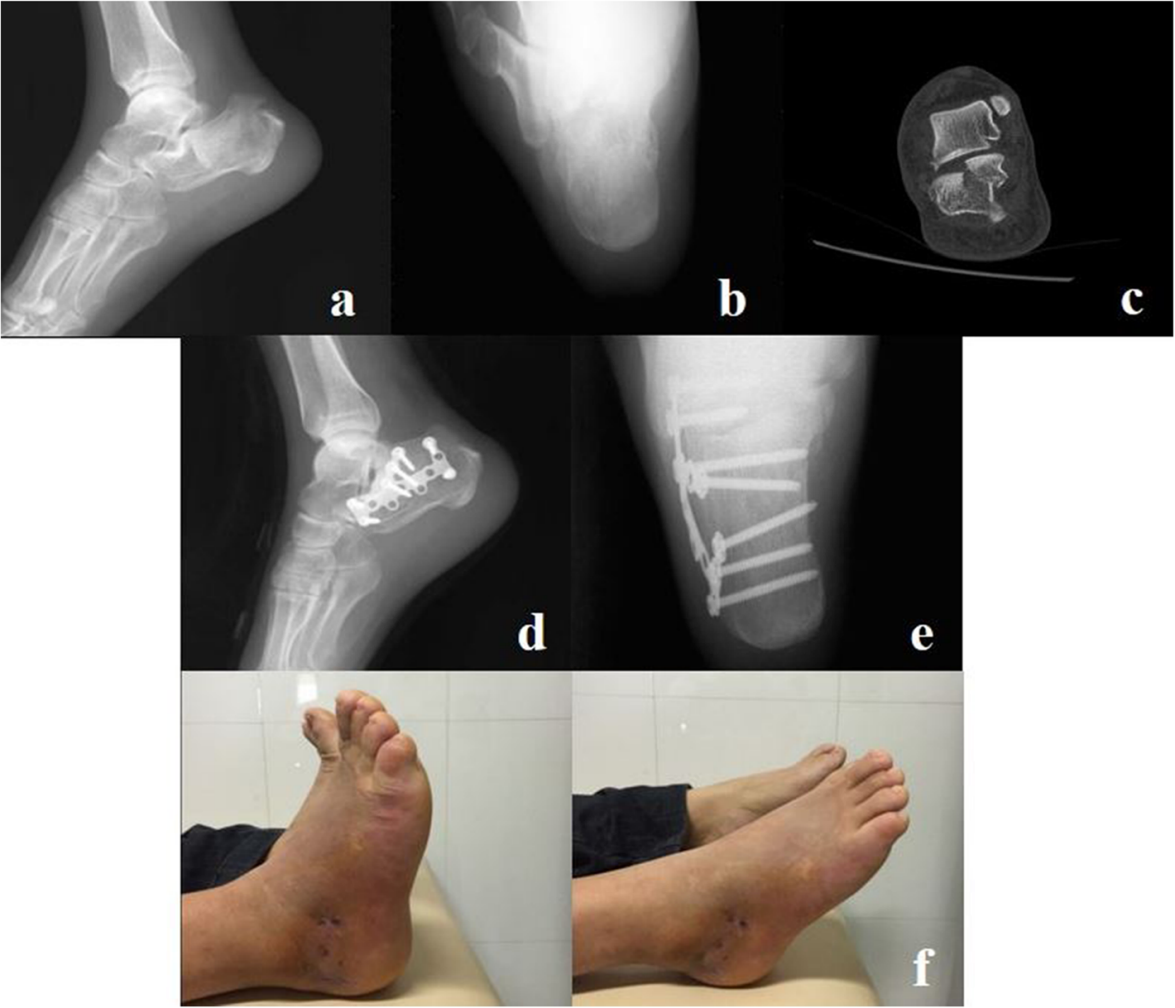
A 36-year-old male patient had a right calcaneal fracture as a result of an accidental fall. a Preoperative lateral radiography. b Preoperative axial radiography. c Preoperatively, the CT scan indicated a Sanders type II fracture. d Postoperative lateral radiography. e Postoperative axial radiography. f Two weeks postoperatively, the motion of the ankle and subtalar joints was almost identical to that of the contralateral limb
